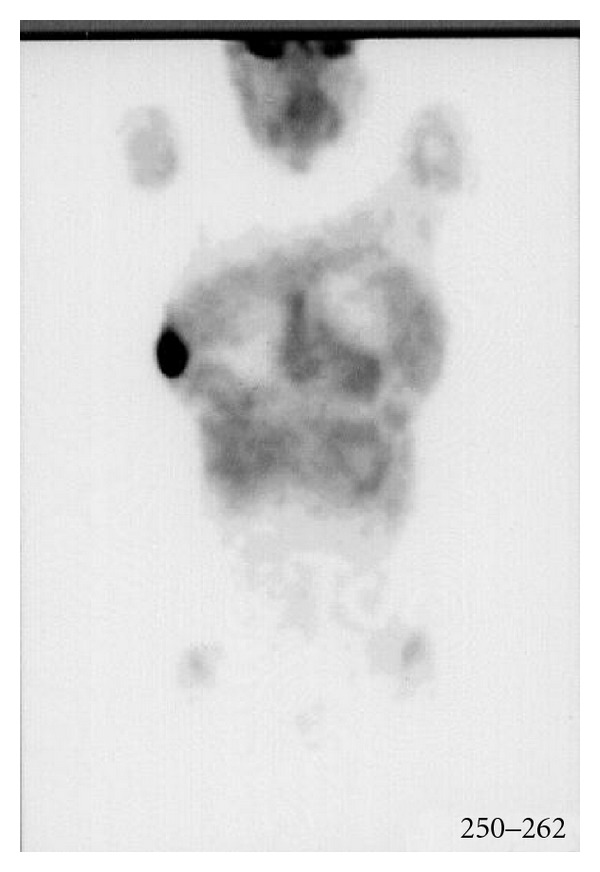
FDG-PET: positive uptake of radiotracer in the right breast.
